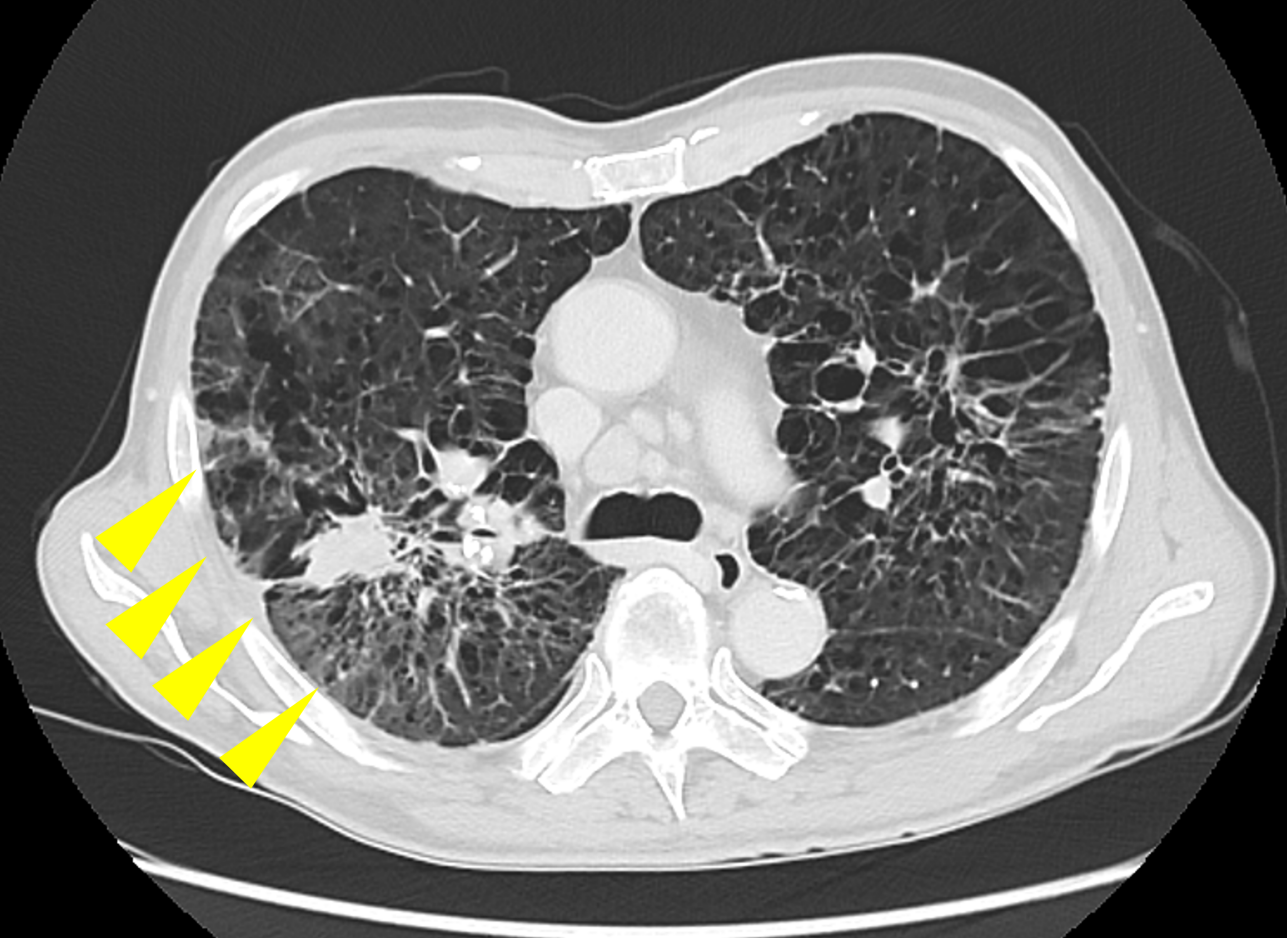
Chest computed tomography scan demonstrates a consolidation and ground‐glass opacity surrounding silicotic nodules (yellow arrow).
